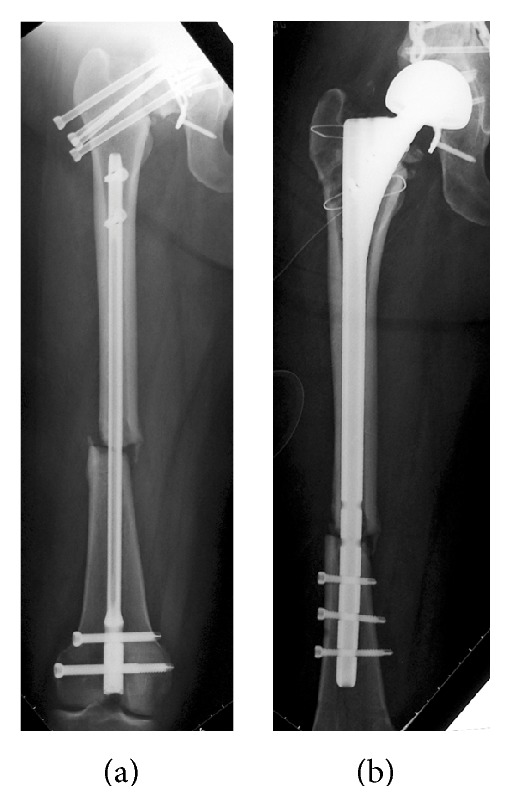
Radiographs of the right femur after the first two operations and after arthroplasty. (a) At 15 weeks after the first two operations, the screws at the femoral neck are loose with nonunion of the femoral neck and shaft. (b) A radiograph showing that arthroplasty was successfully performed.
